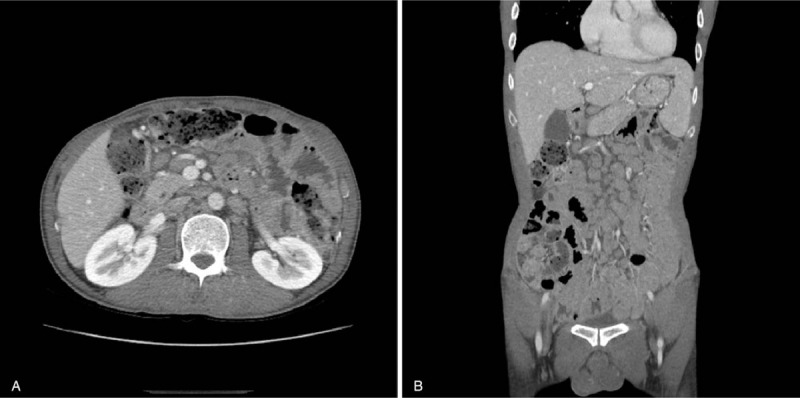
Contrast-enhanced abdominopelvic axial (A) and coronal (B) computed tomography (CT) scans of the case. (A-B) Numerous, small, homogenous, hypovascular lymph nodal enlargement visible in the paraaortic, mesenteric, and both inguinal areas.
